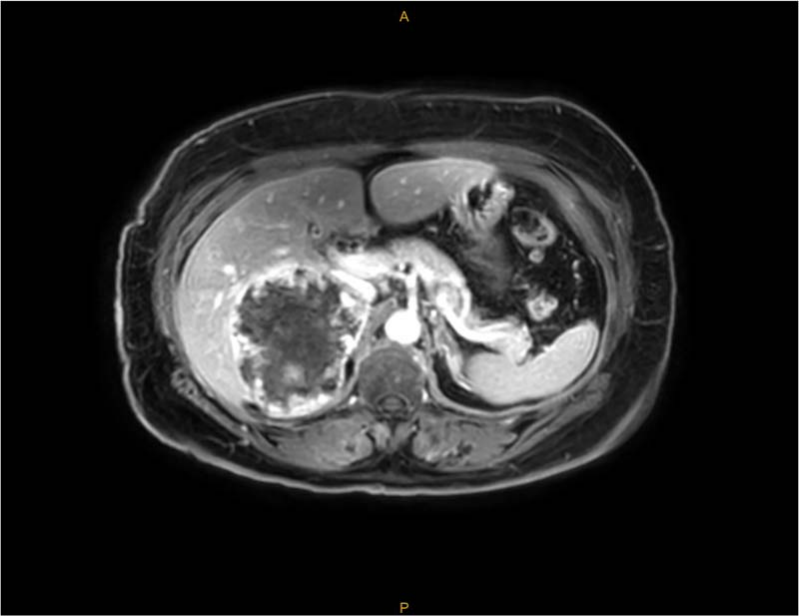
MRI—demonstrating peripheral enhancement in large, complex right-sided adrenal lesion (9.8 × 8.2 × 8.1 cm).
